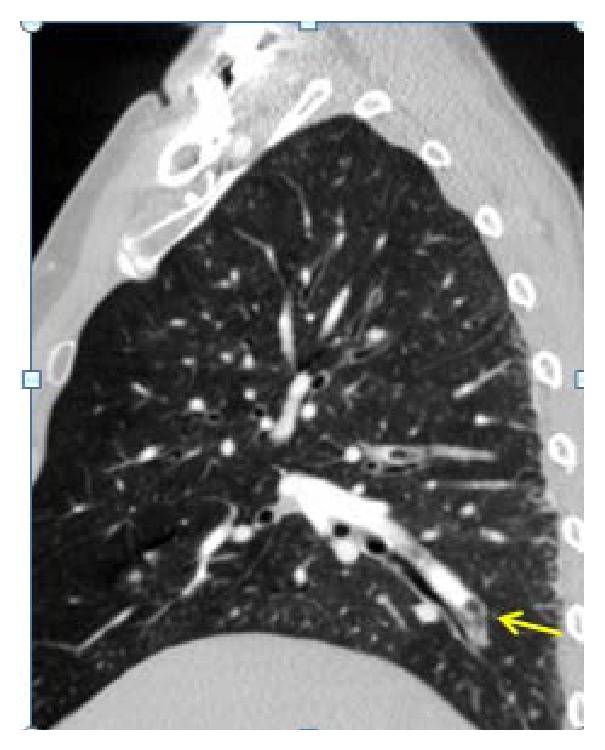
Contrast enhanced sagittal chest CT. Intravascular filling defect with air density bubble in subsegmental artery (yellow arrow) of apical segment of right lower lobe, bronchus is underneath.
